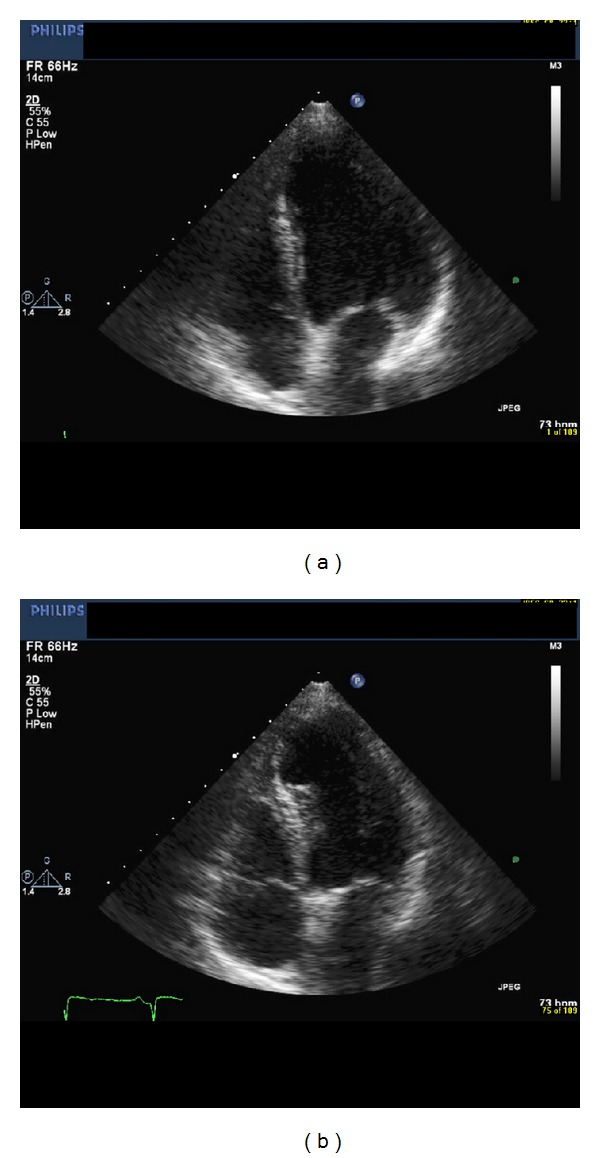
Two-dimensional echocardiographic view demonstrating new anterior wall motion abnormality (apical 4-chamber view) at diastole (a) and systole (b).
